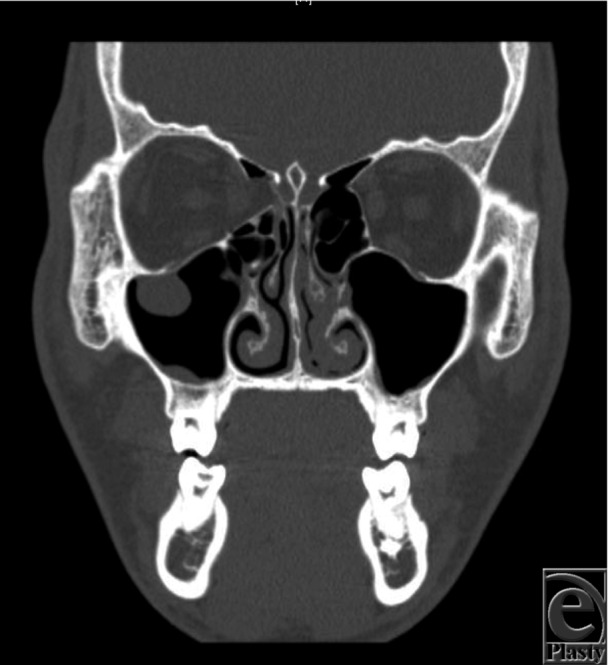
Coronal view of computed tomography max/face revealing a right-sided medial orbital wall blowout fracture without entrapment.
